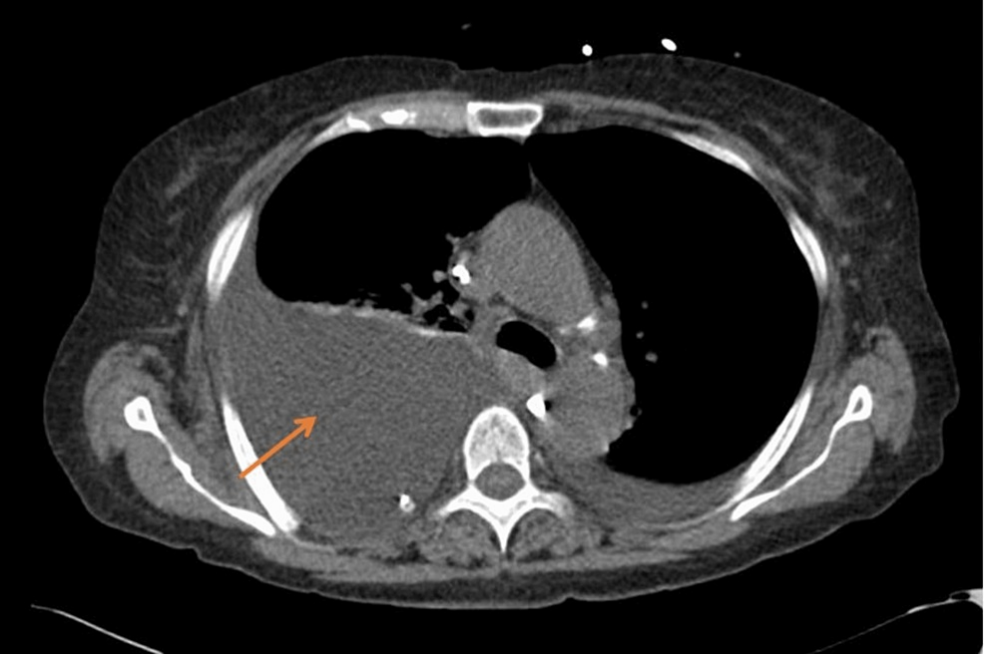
CT scan of the chest on admission CT scan of the chest showing a large right-sided pleural effusion with compressive atelectasis (orange arrow) and a small left-sided pleural effusion
